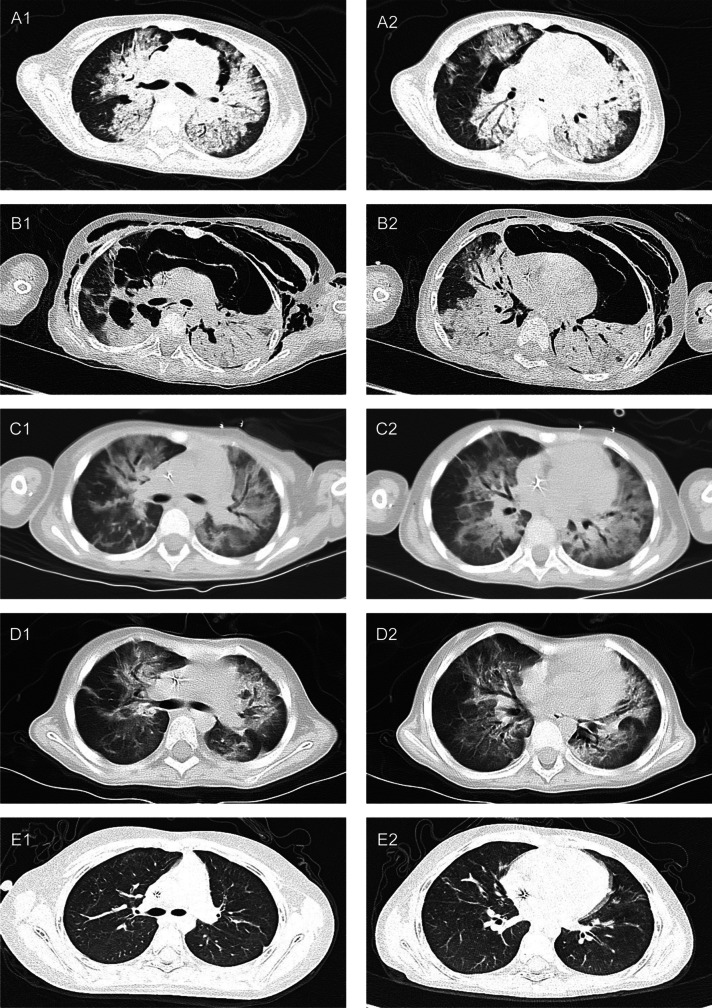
Chest CT results during the course of the disease. Chest CT performed on January 7, 2020(VDLP D23) (A1, A2), on January 8, 2020(VDLP D24) (B1, B2), on January 17, 2020(VDLP D33) (C1, C2),on February 1, 2020(D1, D2), and on June 28, 2020(E1, E2)
